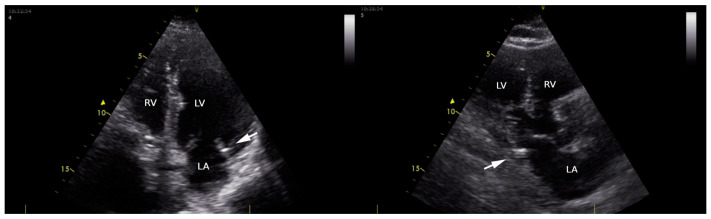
Transthoracic echocardiography in apical four-chamber view (left) and long parasternal view (right), showing mitral annular calcification (arrow) in an enrolled patient. LA: left atrial; LV: left ventricle; RV: right ventricle.
